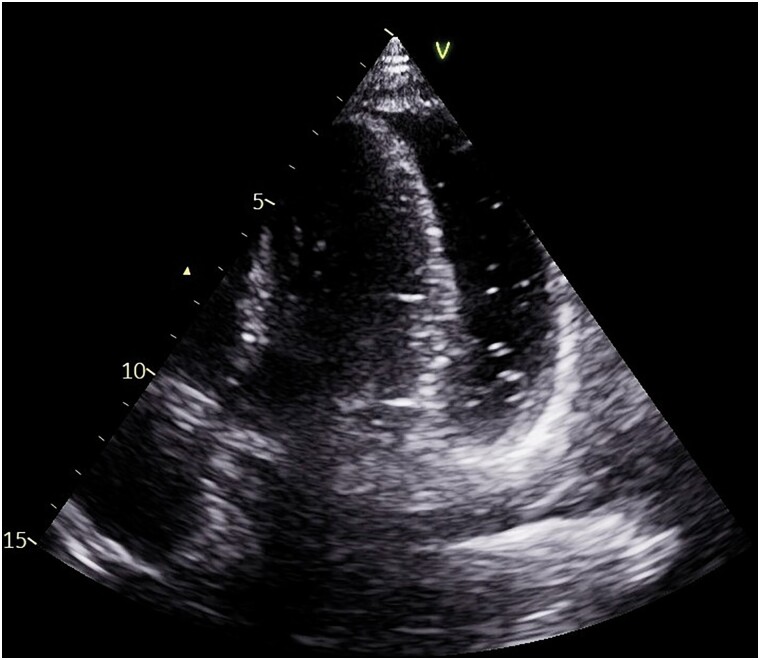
Apical four-chamber findings in pneumohydropericardium. Apical four-chamber view showing moderate pericardial effusion with clear visualization of air bubbles in front of the left ventricular anterolateral wall.
